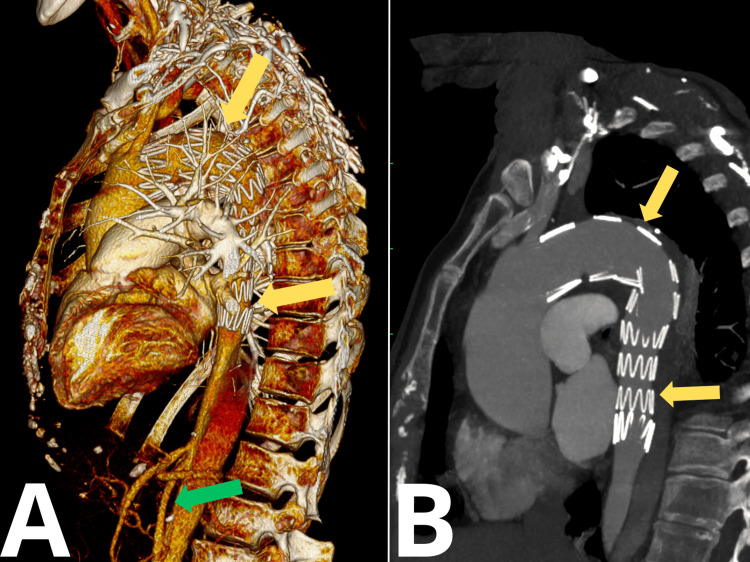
CT During Follow-Up: (A) 3D Reconstruction and (B) Sagittal 2D Plane The yellow arrow indicates the stent graft. The green arrow indicates the patent left common iliac artery CT, computed tomography; 3D, three-dimensional
